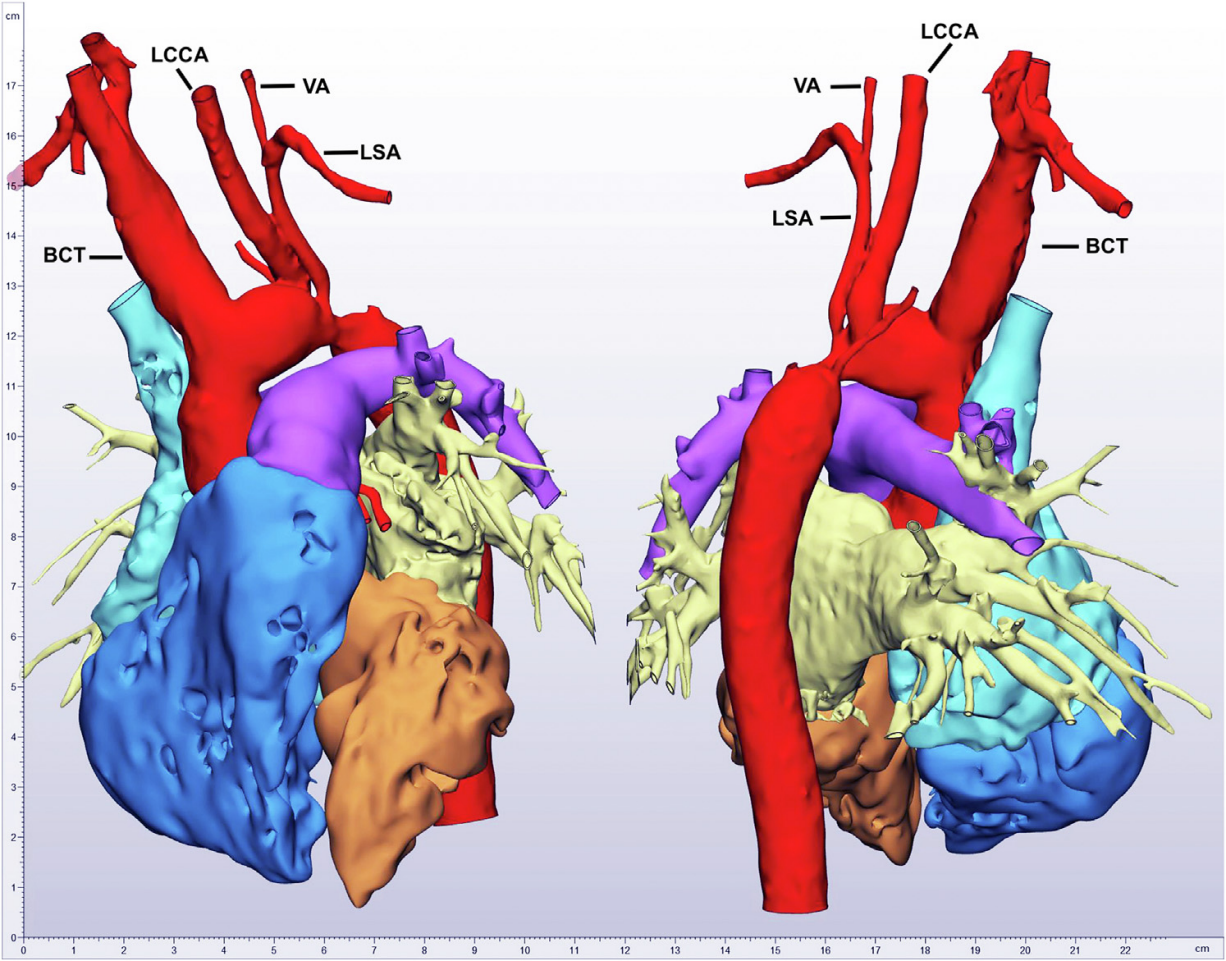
Three-dimensional-model reconstruction of preoperative computed tomography angiography. Abbreviations: BCT, brachiocephalic artery; LCCA, left common carotid artery; LSA, left subclavian artery; VA, vertebral artery.
